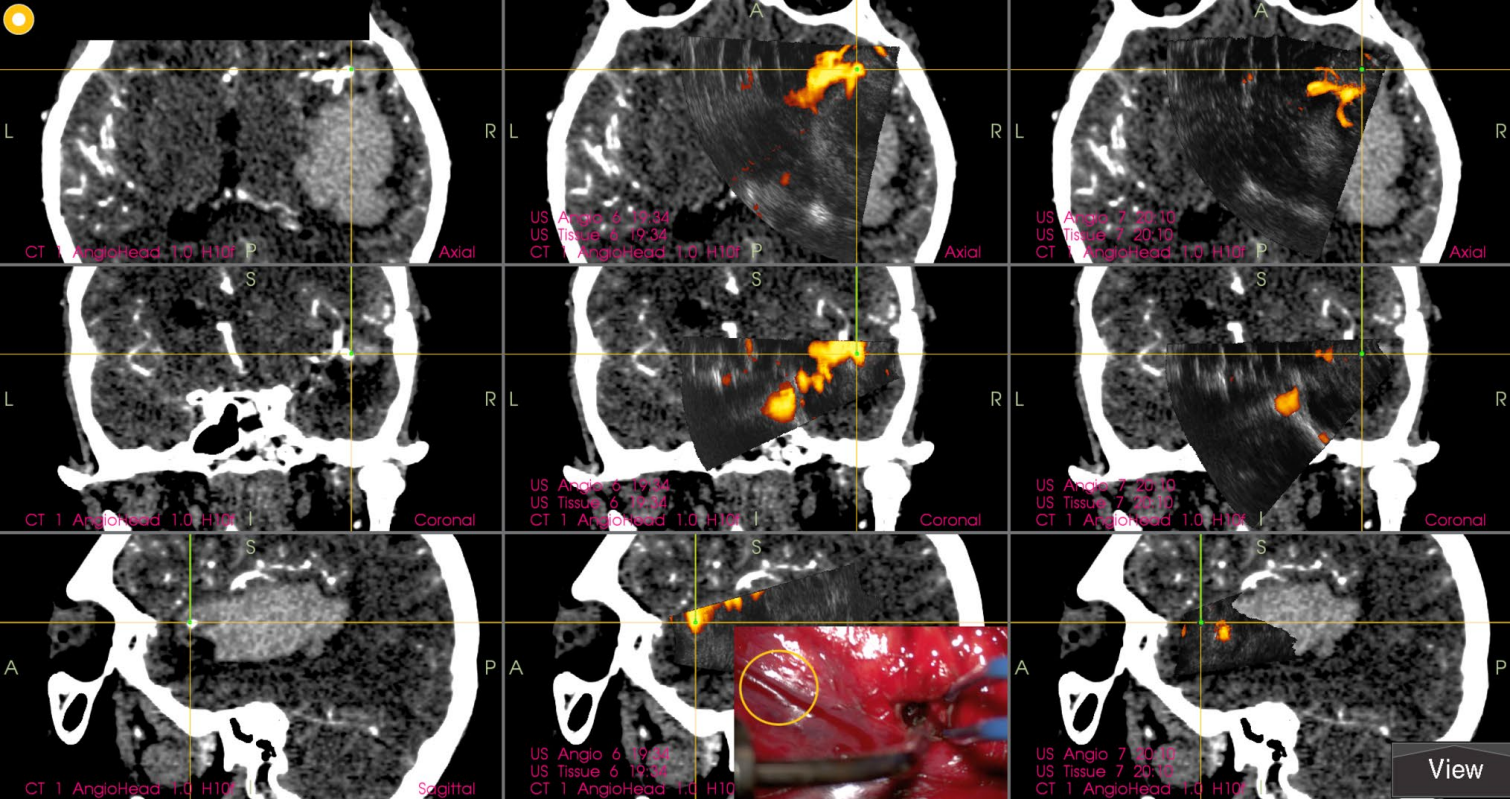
3D-US images of case No. 10. Left column:Preoperative navigation CT angiography. Middle column:Initial 3D-US power-Doppler image acquired before the start of hematoma evacuation, note the aneurysm is well visible in the same place as depicted by preoperative CT. *Inset:*After acquisition of initial 3D-US scans and localization of the (dangerous) area in the vicinity of the aneurysm, partial hematoma evacuation at a safe distance was performed C).  Right column: 3D-US power-Doppler image acquired after partial hematoma evacuation, note the significant shift of the aneurysm. The updated 3D-US image allowed identification of aneurysm position and helped to prevent hematoma evacuation too close to the aneurysm and rebleeding
